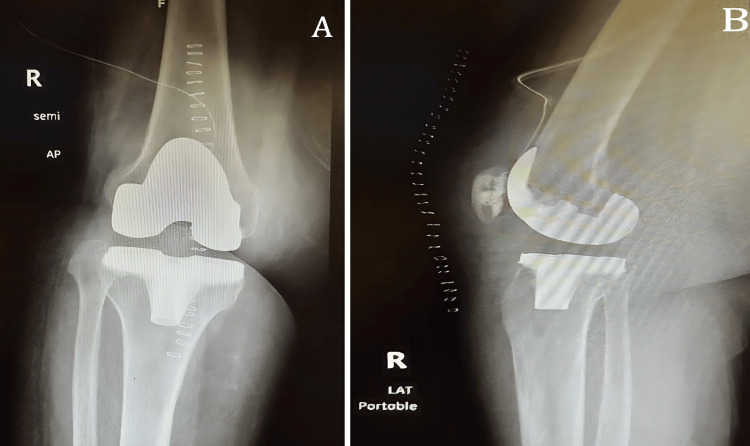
Right knee X-rays immediately postoperatively: (A) AP view and (B) lateral view AP: anteroposterior
